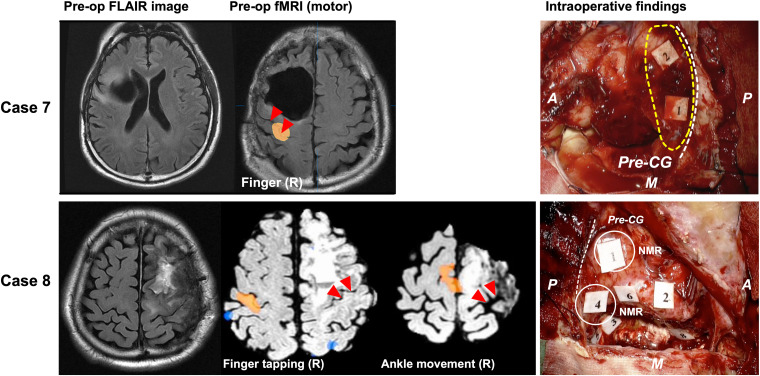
Patients whose motor functioning shifted, but the destination of the shift is unknown. The left column shows the preoperative FLAIR image. In case 8 (lower column), the functional MRI investigation for the movement task of the right finger and the foot revealed the BOLD in the precentral and postcentral gyri of the right cerebral hemisphere (middle two columns). During surgery, involuntary movement was not observed in the motor area, but negative motor response was observed (Right lower column, white circle). The red triangles in the middle column and white broken line in the right column indicate the central sulcus; The yellow broken line in the upper right column indicates motor area recognized as positive motor response in previous surgery; A: anterior; P: posterior; M: medial side; broken white line: central sulcus. Tags indicate intraoperative findings as follows: Case 7: Tags 1 and 2, anarthria; Case 8: Tags 1 and 4, negative motor response (NMR) in cortical level; Tag 2, paraphasia; Tags 3 and 5, NMR in subcortical level; Tags 6 and 8, paraphasia; A: anterior; P: posterior; M: medial side.
